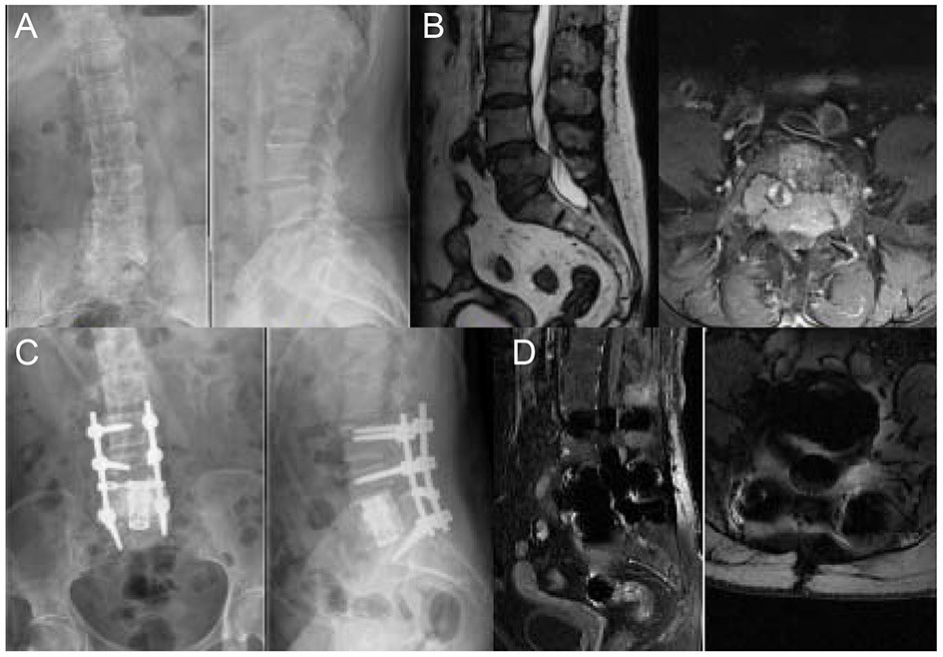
A 59 years old woman with breast cancer bone metastasis in L5; Her preoperative Frankel grade was D. (A) Preoperative anteroposterior and lateral radiograph revealed uneven density in L5. (B) Preoperative sagittal T2-weighted and axial T1-weighted MRI revealed the metastatic lesion with an intraspinal lesion. The protrusion of soft tissue resulted in compression of the dura and canal stenosis. (C) Postoperative anteroposterior and lateral radiograph showed that posterior spinal fusion with anterior reconstruction was performed. (D) Postoperative sagittal T1-weighted MRI.
